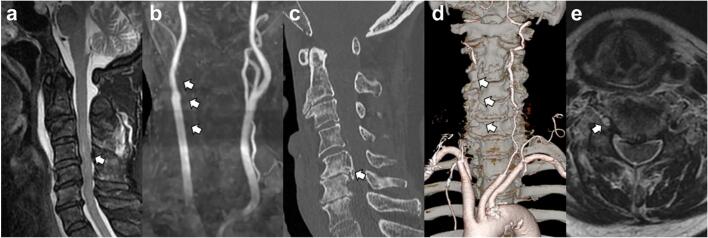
Imaging findings of the cervical spine and vertebral artery occlusion. (a) Sagittal T2-weighted fat-suppressed MRI demonstrating hyperintensity from C4 to C7, consistent with spinal cord edema and posterior soft tissue injury (arrow). (b) MRA revealing complete occlusion of the right vertebral artery (arrow). (c) Sagittal non-contrast CT depicting cervical canal stenosis due to the ossification of OPLL (arrow). (d) Contrast-enhanced CT confirming occlusion of the right vertebral artery (arrow). (e) Axial T2-weighted fat-suppressed MRI at the C5 level showing a normal flow void in the left vertebral artery and loss of the expected flow void in the right vertebral artery, suggestive of thrombosis (arrow).
